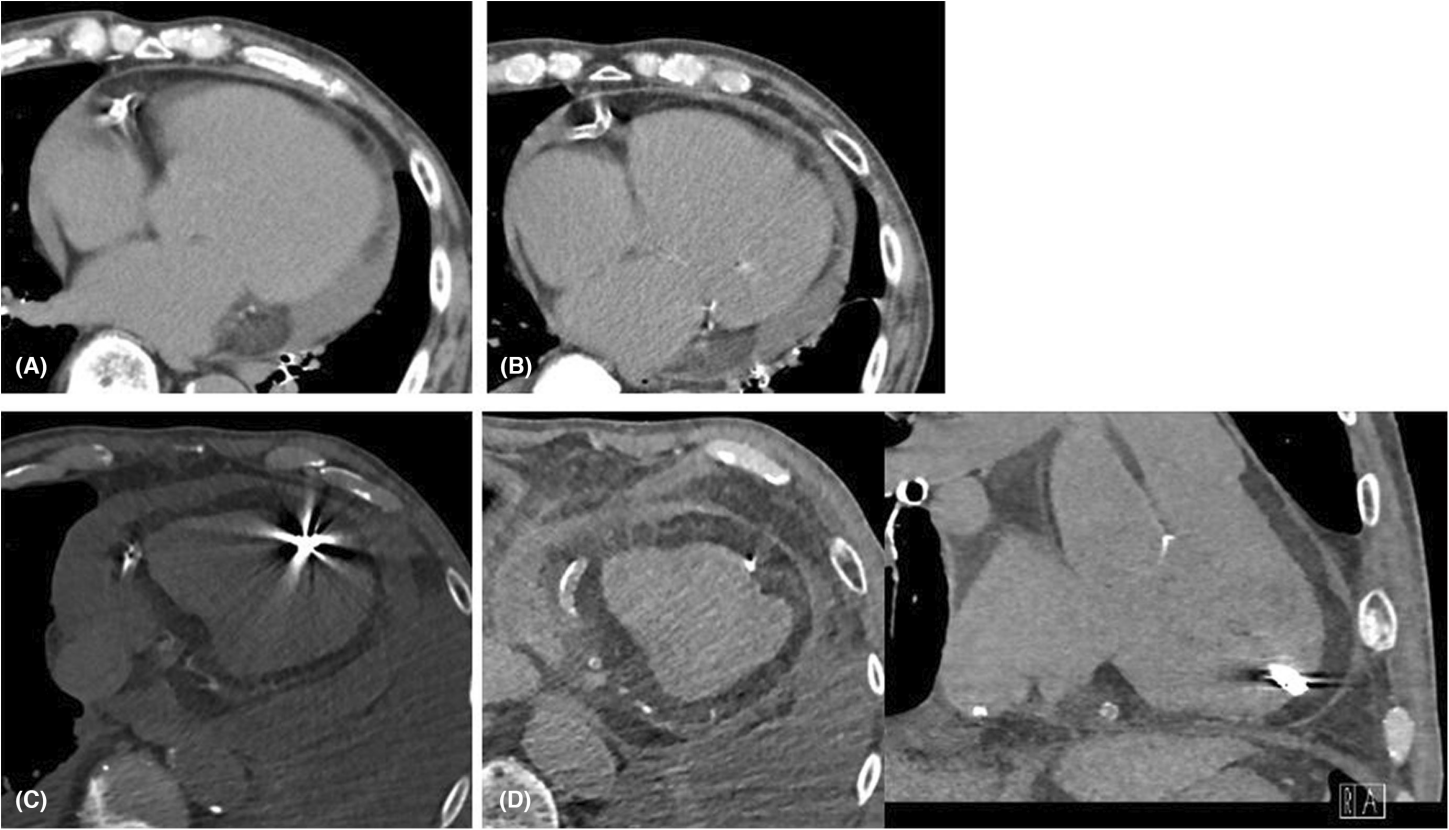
(A) Computed tomography (CT) image before leadless pacemaker implantation (LPI). CT shows pericardial effusion of unknown cause mainly in the posterior left ventricle. (B) CT image of the LP 1 month after LPI. (C) The LP at re‐admission of late cardiac tamponade. (D) The LP tip after pericardial drain removal (left panel; axial view; right panel, coronal plane).
